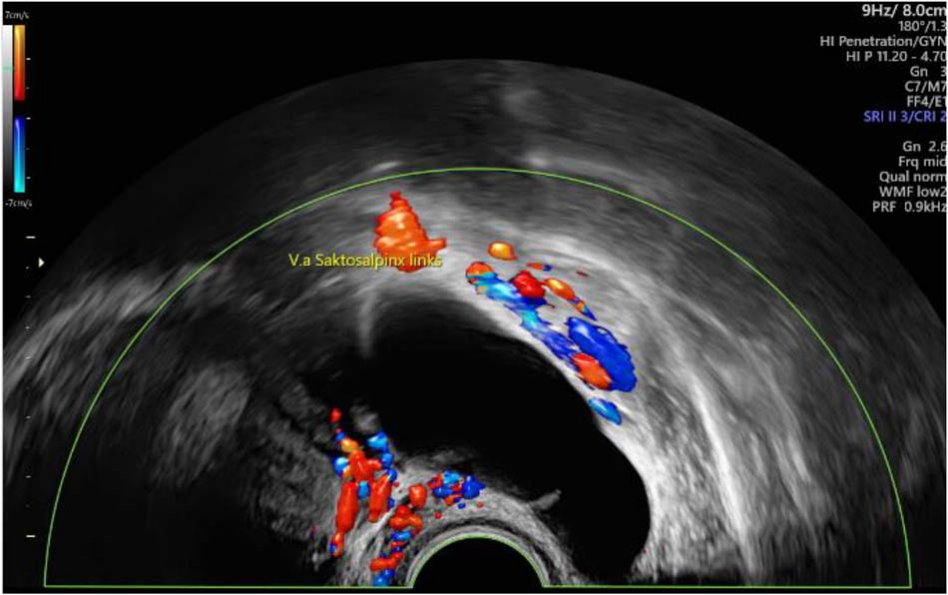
Preoperative sonographic suspicion of hydrosalpinx on the left side (64 × 26 mm^2^, echo-poor with dorsal sound enhancement).
